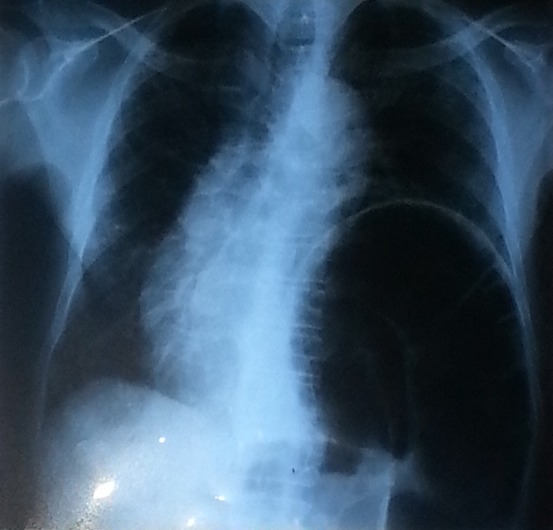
Radiographie de thorax de face complétant l’abdomen sans préparation montrant l’ascension de la coupole diaphragmatique gauche avec l’image en arceau et la dextrocardie
